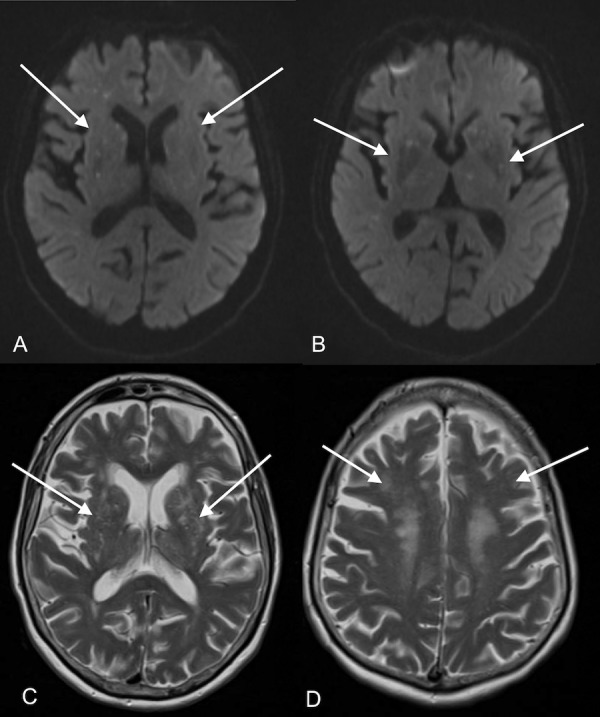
Magnetic resonance imaging (MRI) Diffusion-weighted (DW) MRI. (A, B) demonstrates multiple punctate foci of diffusion restriction in the gray-white junction; T2-weighted axial MRI (C, D) shows bilateral hyperintense areas in the periventricular white matter. Obtained six days after right hip arthroplasty.
